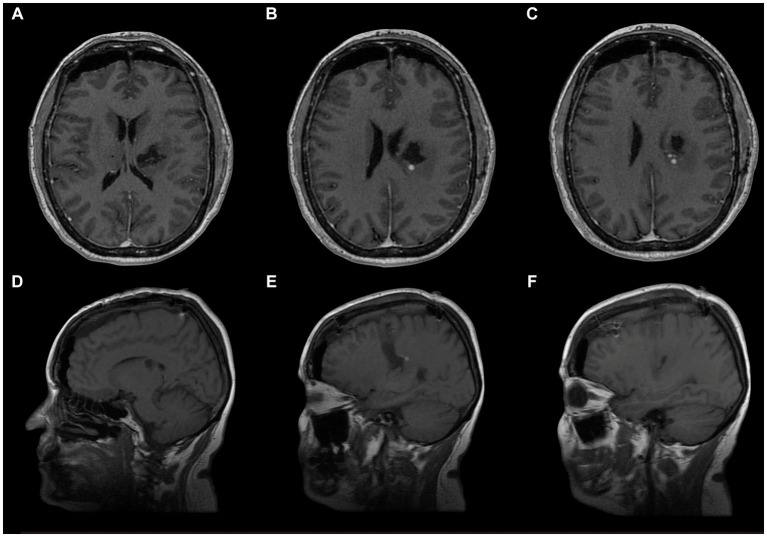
Postoperative MRI of the surgical resection of the cavernoma of the posterior limb of the internal capsule. (A–C) Axial view. (D–F) Sagittal view. Note the hypointense image suggesting complete resection of the cavernoma and structural indemnity of the adjacent anatomy.
